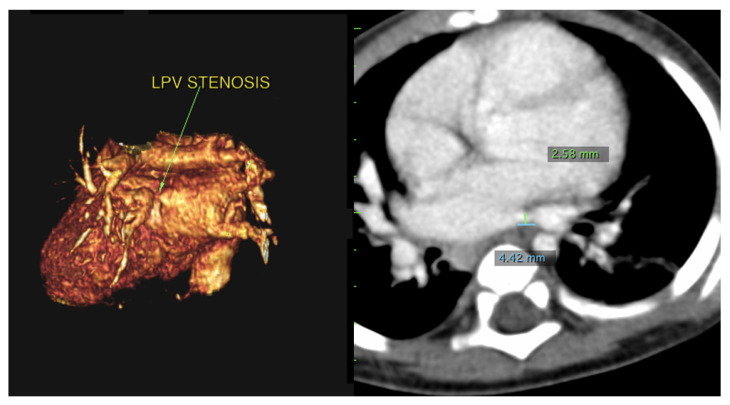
CT scans from the above described patient from Vietnam with left common pulmonary vein stenosis. The left image is a 3D reconstruction of a CT angiogram with the view of the left pulmonary vein connection to the left atrium. The arrow denotes the area of stenosis. The right image is a traditional CT angiogram with sternum anterior and spine posterior. The green marker is of the stenosis which narrows to 2.58 mm. The blue marker is of the length of the area of stenosis which is 4.42 mm.
